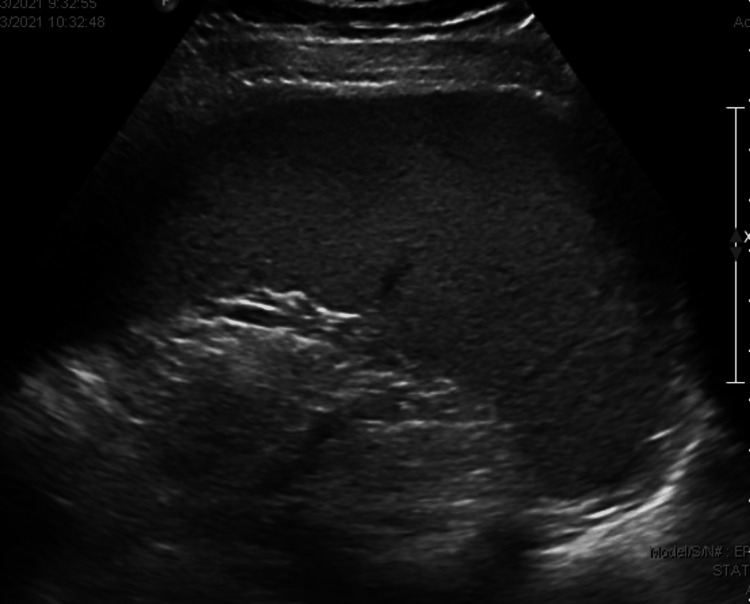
Abdominal ultrasound revealing mild splenomegaly
